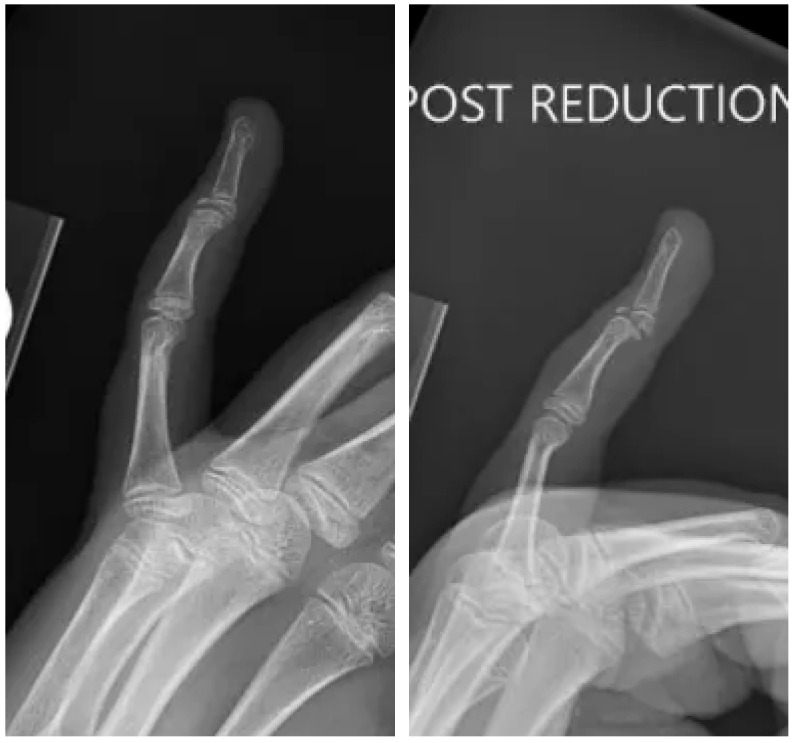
Post-reduction radiographs. Oblique and lateral views of the small finger in a skeletally immature individual demonstrating a reduced distal phalanx with comminuted intra-articular fracture.
